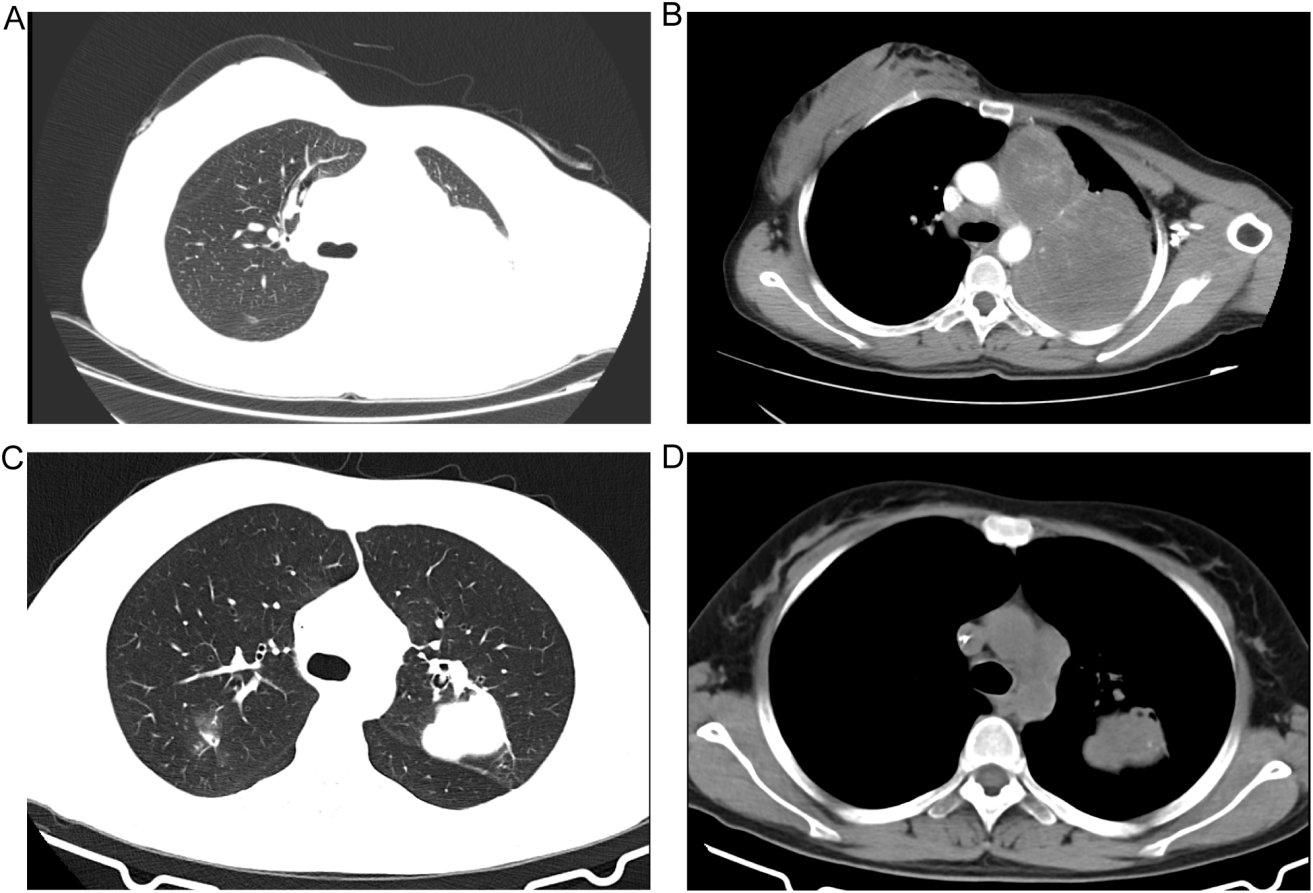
Comparison of chest CT scans before and after neoadjuvant chemotherapy. Pre-treatment contrast-enhanced CT scans showing the primary tumor in the (A) lung window and (B) mediastinal window. Post-treatment non-contrast CT scans after 6 cycles of neoadjuvant chemotherapy (ifosfamide + epirubicin regimen) showing tumor response in the (C) lung window and (D) mediastinal window.
